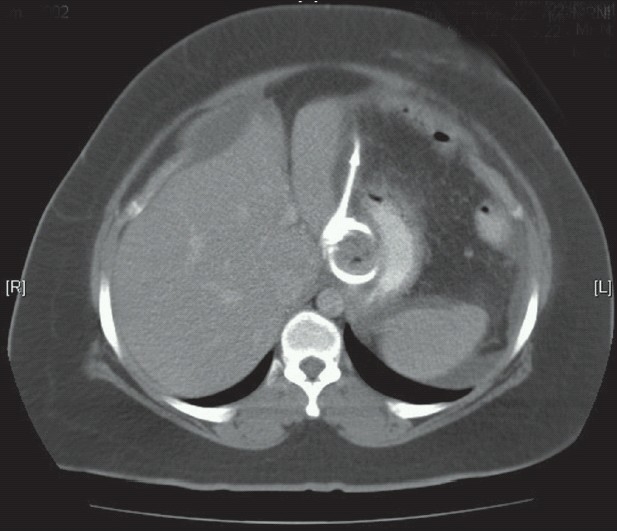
CT scan of the abdomen showing an enlarged liver with a gastric band *in-situ*
